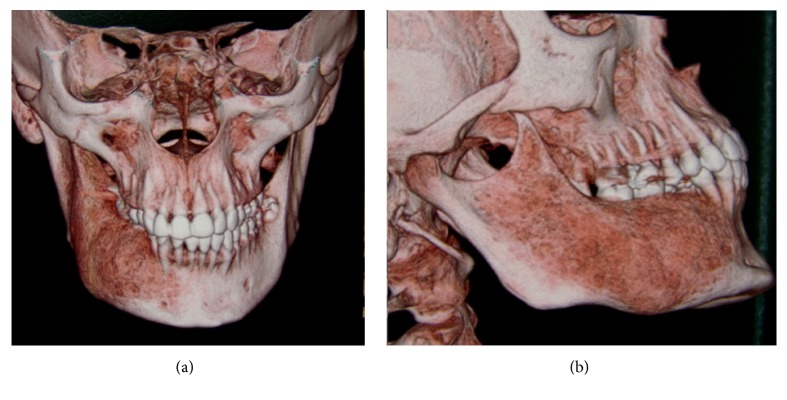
3D reconstruction by cone-beam computed tomography showing an increase in the volume of the right hemimandible. (a) Anterior view and (b) lateral view.
